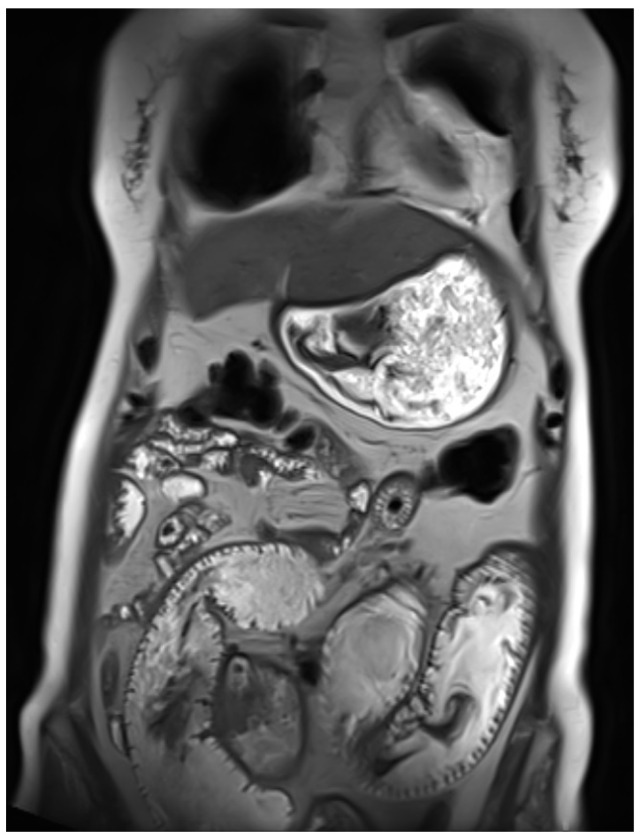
Dilation of intestinal loops on MRI.
